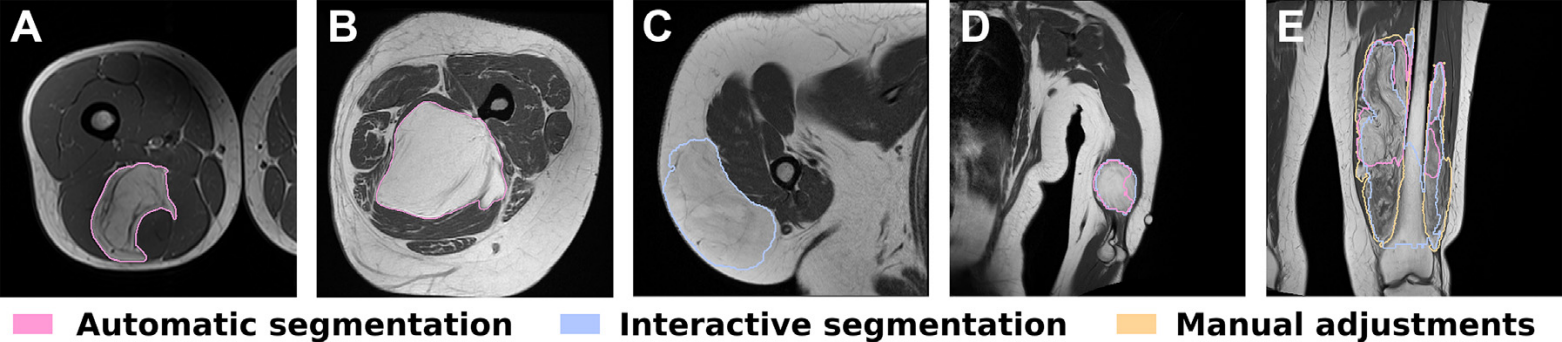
Examples of automatic, interactive and manual segmentations on T1-weighted MRI images. In image A and B, the automatic segmentation method achieves excellent alignment. However, in image C and D, the automatic segmentation method did not result in any segmentation (C) or proved to be insufficient (D) and required interactive segmentation to obtain satisfactory results. Finally, in image E, manual adjustments were required to refine the segmentation after unsatisfactory outcomes from both automatic and interactive approaches in the region of interest. For visualization purposes, 2D slices are shown, however, all data are 3D images.
